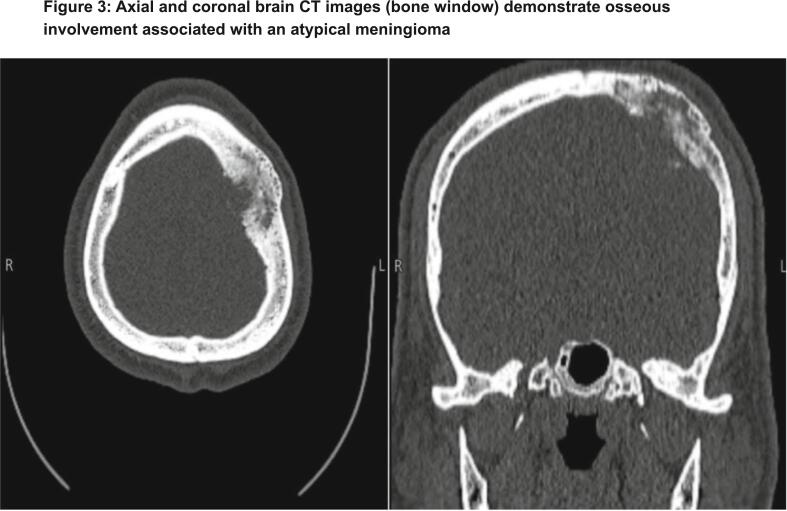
Axial and coronal brain CT images (bone window) demonstrate osseous involvement associated with an atypical meningioma.
